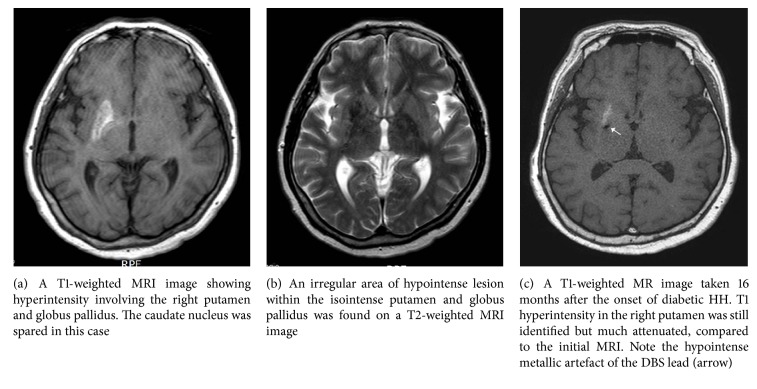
Magnetic resonance imaging (MRI) findings of diabetic hemichorea/hemiballism.
